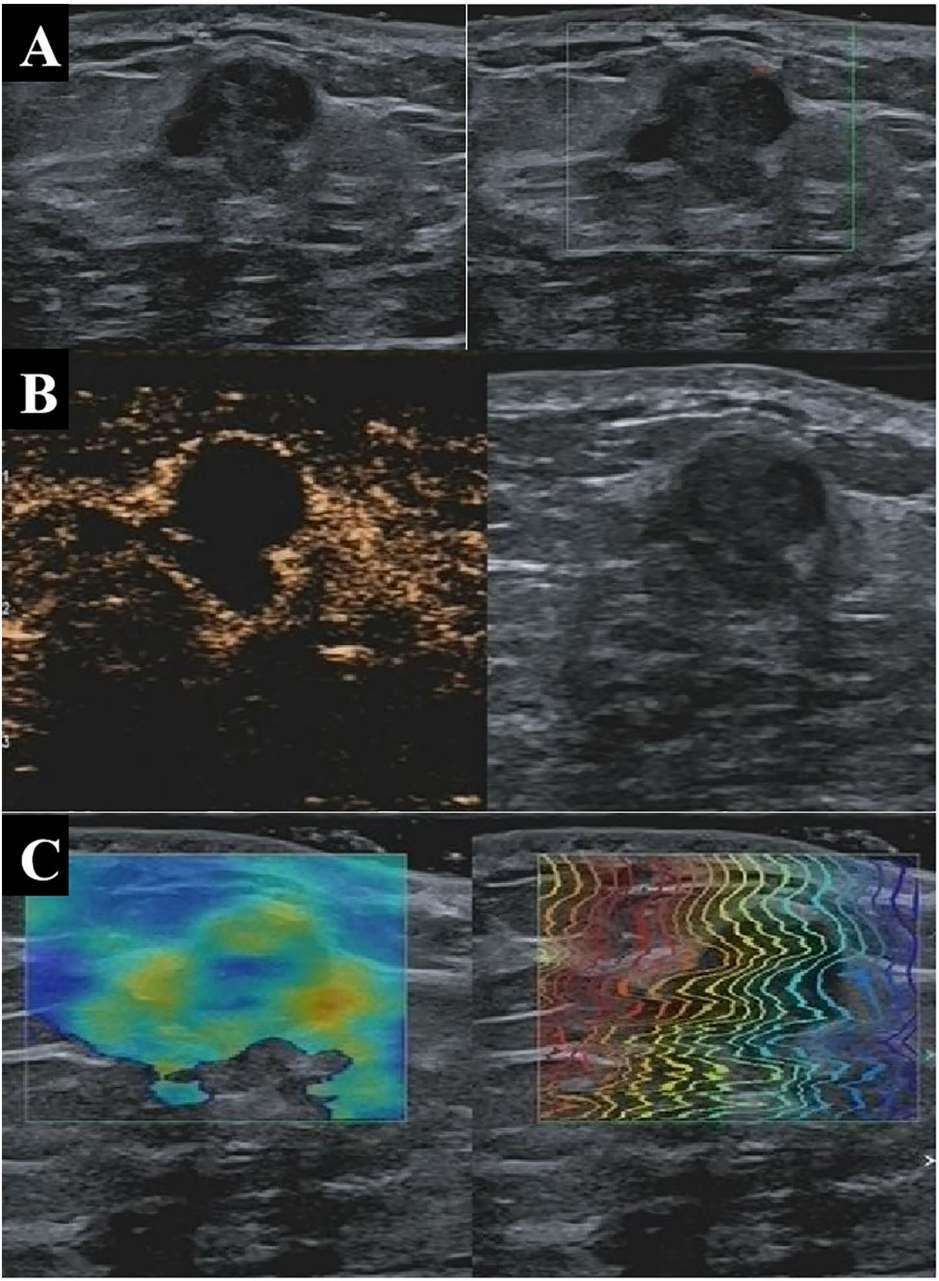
A 33-year-old patient with pathologically confirmed breast adenosis showing a hypoechoic solid nodule at 4 o’clock in the right breast with well-defined margins, irregular shape, and poor blood flow on conventional US (A), indicating a malignant lesion with a BI-RADS 4b score. On CEUS, the tumor appears as a nodule without blood perfusion (B), indicating a benign lesion with a BI-RADS 3 score. The nodule was soft based on shear wave elastography, indicating that the lesion was benign (C). The nodule was assessed as benign by the multi-parameter predictive model. US, ultrasonography; CEUS, contrast-enhanced ultrasonography; BI-RADS, Breast Imaging Reporting and Data System.
